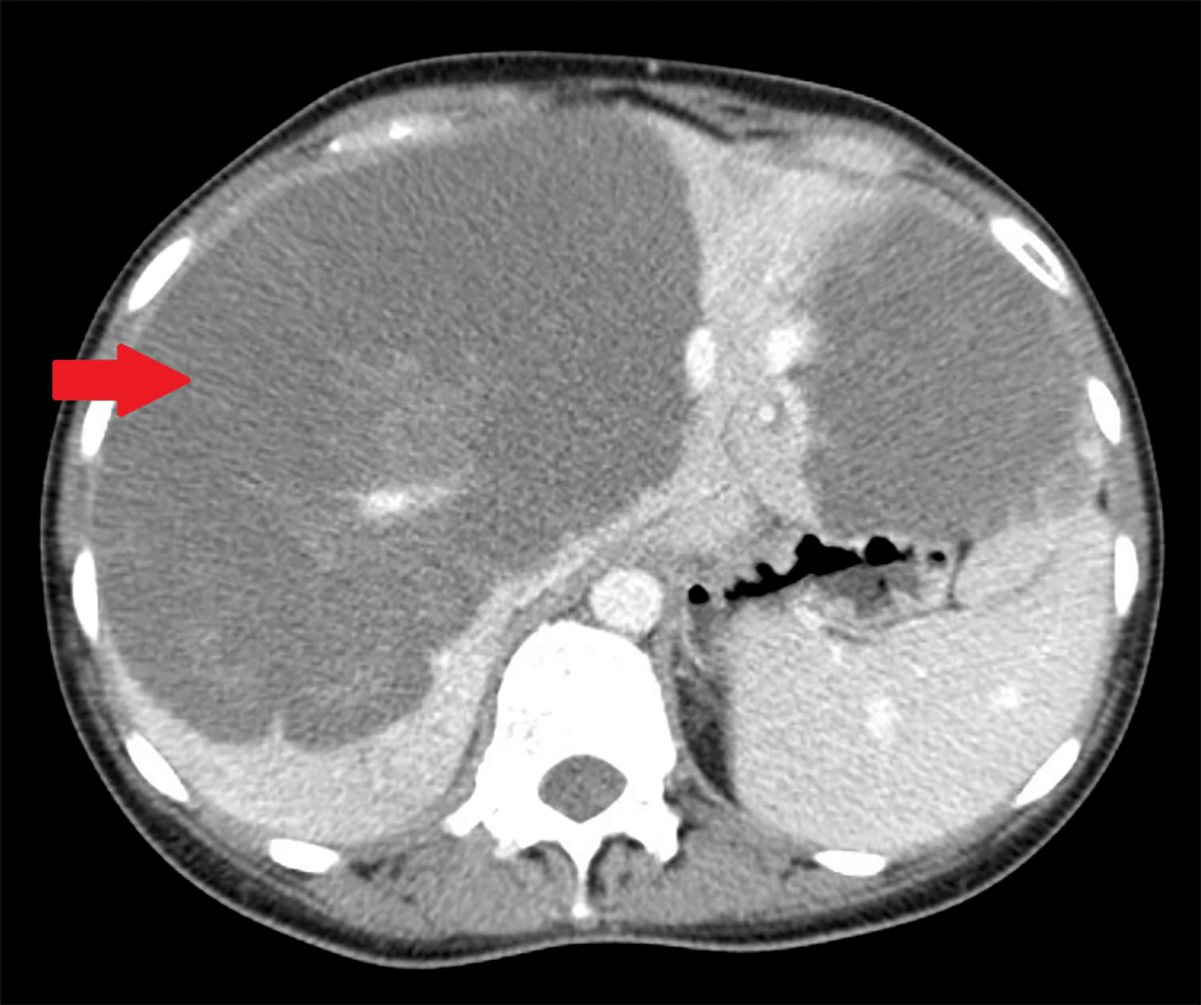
Transverse section of computed tomography (CT) scan of the abdomen and pelvis. The arrow points towards the liver mass.
